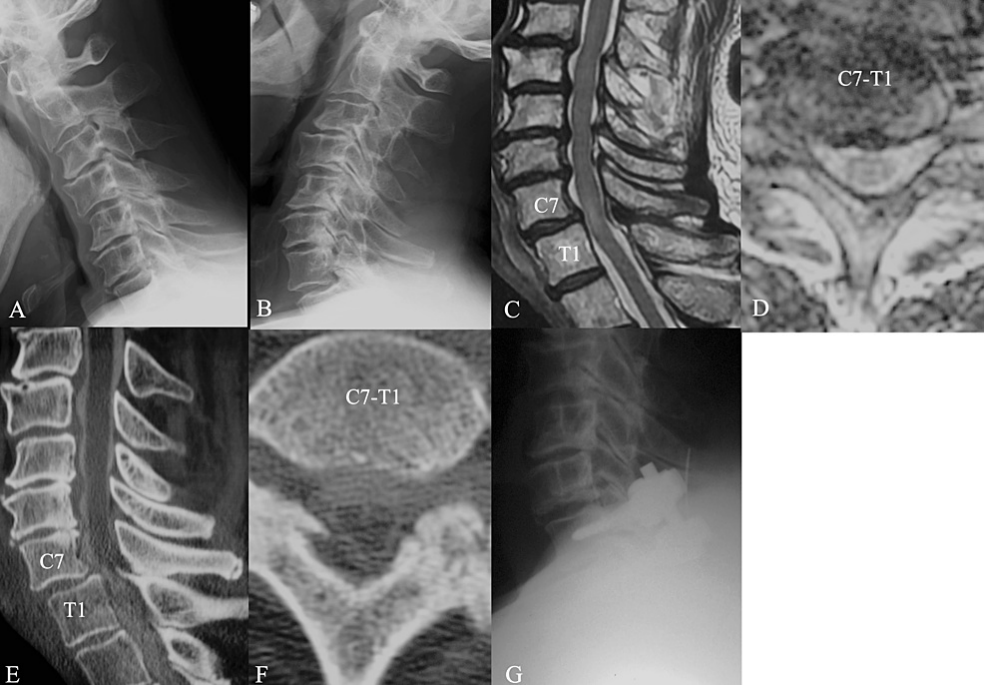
Imaging findings in Case 2 (A) Preoperative cervical spine radiograph in flexion lateral view. C7-T1 level has mild listhesis. (B) Preoperative cervical spine radiograph in extension lateral view. (C) T2-weighted sagittal magnetic resonance imaging (MRI). The spinal cord at the C7-T1 level has high intramedullary intensity. (D) T2-weighted axial MRI at the C7-T1 level, with the spinal cord appearing to be slightly compressed. (E) Paramedian slice of the sagittal computed tomographic myelography (CTM) image showing mild anterior spondylolisthesis at the C7-T1 level and ossification of the ligamentum flavum (OLF) on the right side. (F) Axial CTM view showing OLF compressing the spinal cord. (G) Lateral view of the postoperative radiograph. C7-T1 laminectomy and posterior instrumented fusion were performed.
